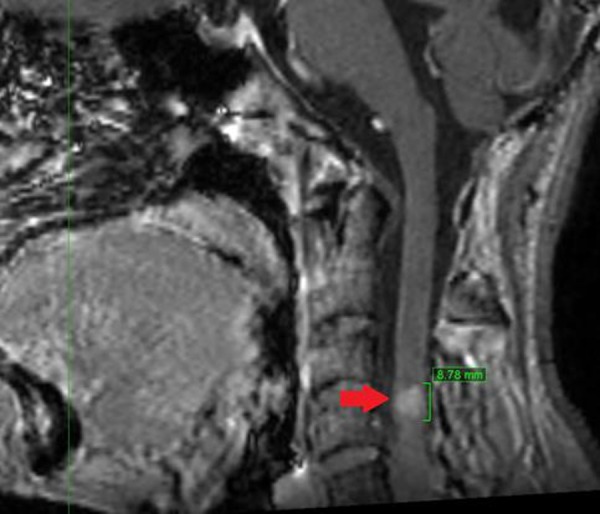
Sagittal view of magnetic resonance imaging, slice thickness 1.2 mm, lesion at C3-C4 level marked with red arrow
